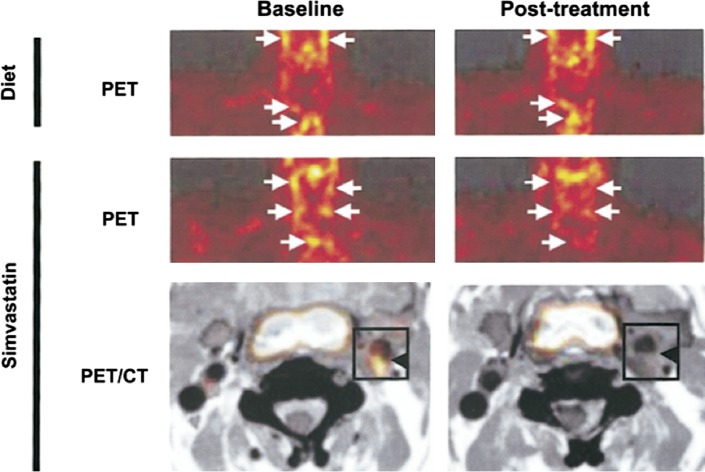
Reduced [18F]FDG following simvastatin therapy. Representative images of a patient on dietary management alone (*top row*). Three-months of dietary management alone had no impact upon FDG uptake in aortic and carotid vasculature (*arrows*). However, FDG uptake is visibly reduced in the carotid arteries and aortic arch following 3 months of therapy with simvastatin (*middle row*). Hybrid FDG PET/CT images demonstrate that following 3 months of therapy with simvastatin, there is no evidence for visible FDG uptake (*bottom row*) (Reprinted from Ref^94^with permission Elsevier)
